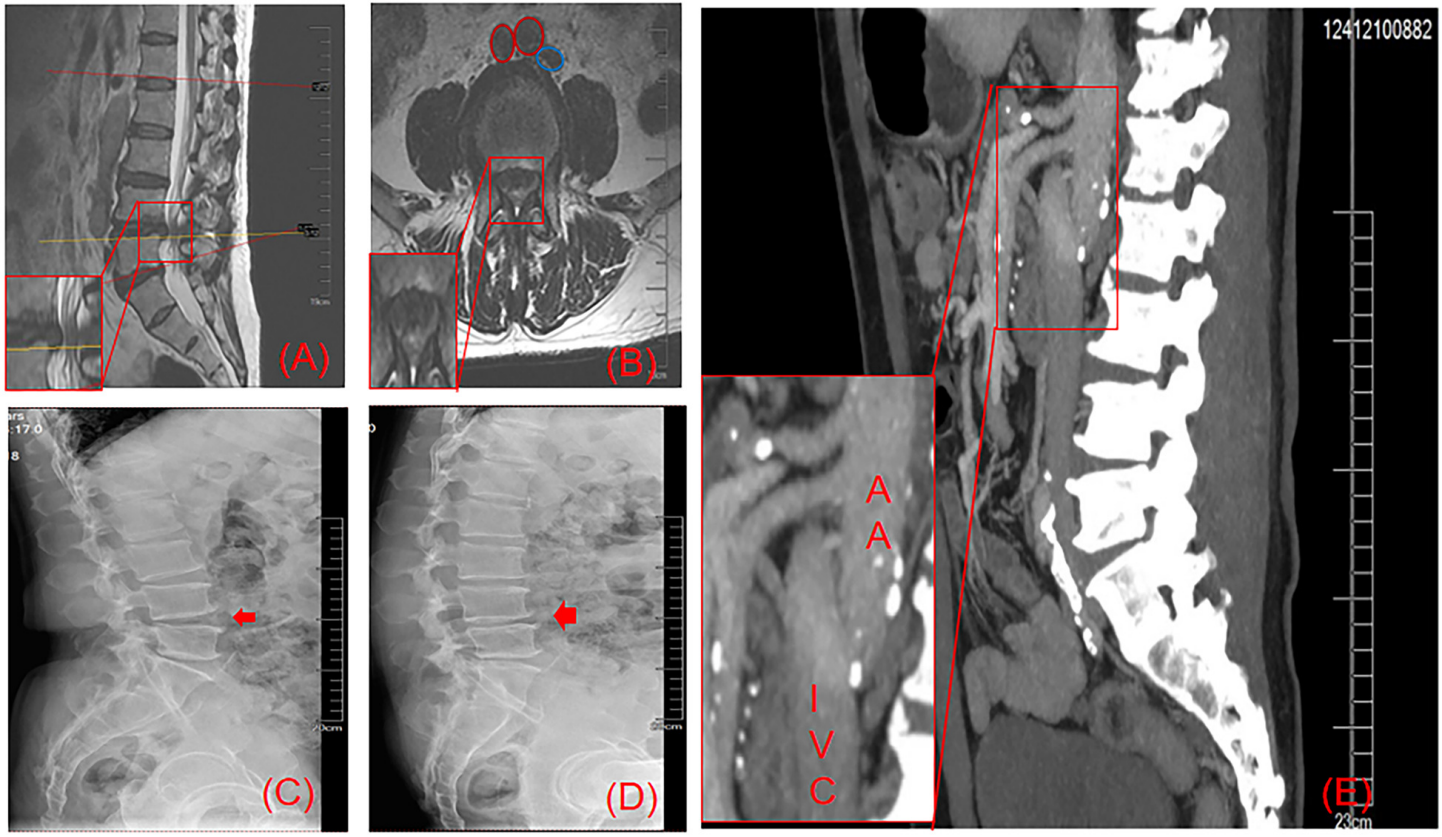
As shown in the magnified sagittal (A) and axial (B) T2-weighted MRI views, a massive L4-5 disc herniation with significant spinal canal stenosis and neural compression is observed. Figure B demonstrates the left-sided IVC (blue circle) and the bifurcated AA (red circle) at the L4-5 level. Comparative dynamic radiographs (C): hyperextension; (D) hyperflexion) reveal 11° of angular change between the L4-5 endplates. (E) (IVC venography) confirms the IVC's left-anterior course along the spine, crossing ventral to the aorta at the L1 inferior endplate.
